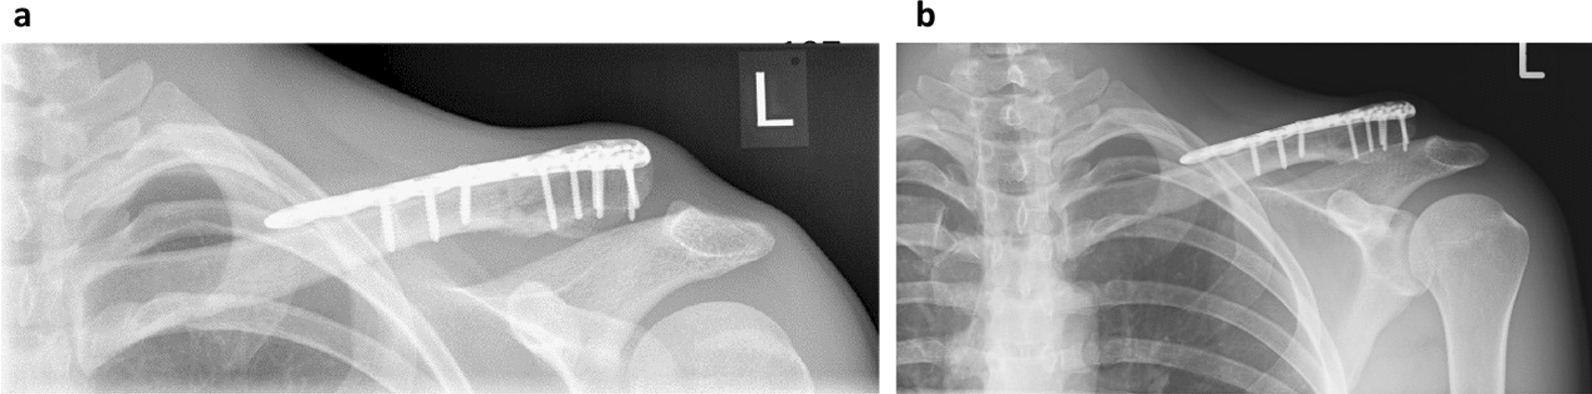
a Radiograph 6 weeks post-surgery. Condition after a lateral clavicle fracture treated with plate osteosynthesis and EMTT stimulation. The foreign material is intact, with no signs of loosening. The fracture gap appears partially healed. b Radiograph 10 weeks post-surgery showing complete bone healing following a clavicle fracture.
